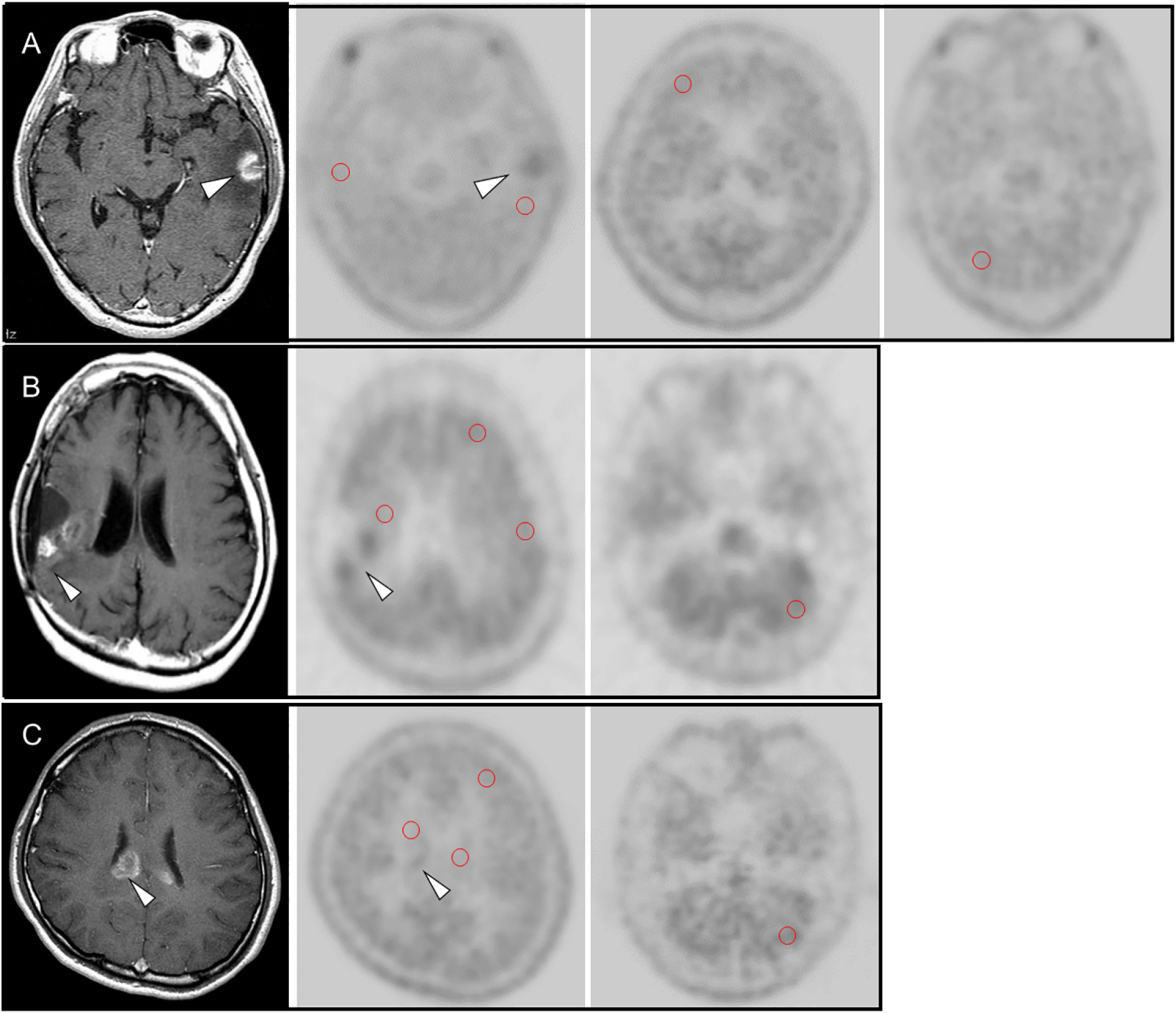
(A) 60-year-old male with recurrence of brain metastasis (lung carcinoma) at left temporal lobe (arrow head). MET uptake of the MRI-enhanced lesion showed higher than the region around the lesion, the contralateral brain, the contralateral frontal lobe and cerebellum. (B) 26-year-old male with recurrence of an anaplastic astrocytoma in the right temporal lobe. Contrast-enhanced MRI showed gadolinium-enhanced nodular lesion (arrow) in the right temporal lobe, which was the location of post-surgical resection of the primary brain tumor. MET uptake of the MRI-enhanced lesion (arrow head) showed higher than the region around the lesion, the contralateral brain, the contralateral frontal lobe and cerebellum. (C) 59-year-old female with radiation necrosis. Contrast-enhanced MRI showed gadolinium-enhanced lesion (arrow head) at the corpse callosum, which was suspected recurrent brain metastasis (lung carcinoma). MET uptake of the MRI-enhanced lesion showed slightly higher than the region around the lesion, the contralateral brain, and the contralateral frontal lobe, but similar to MET uptake at cerebellum.
